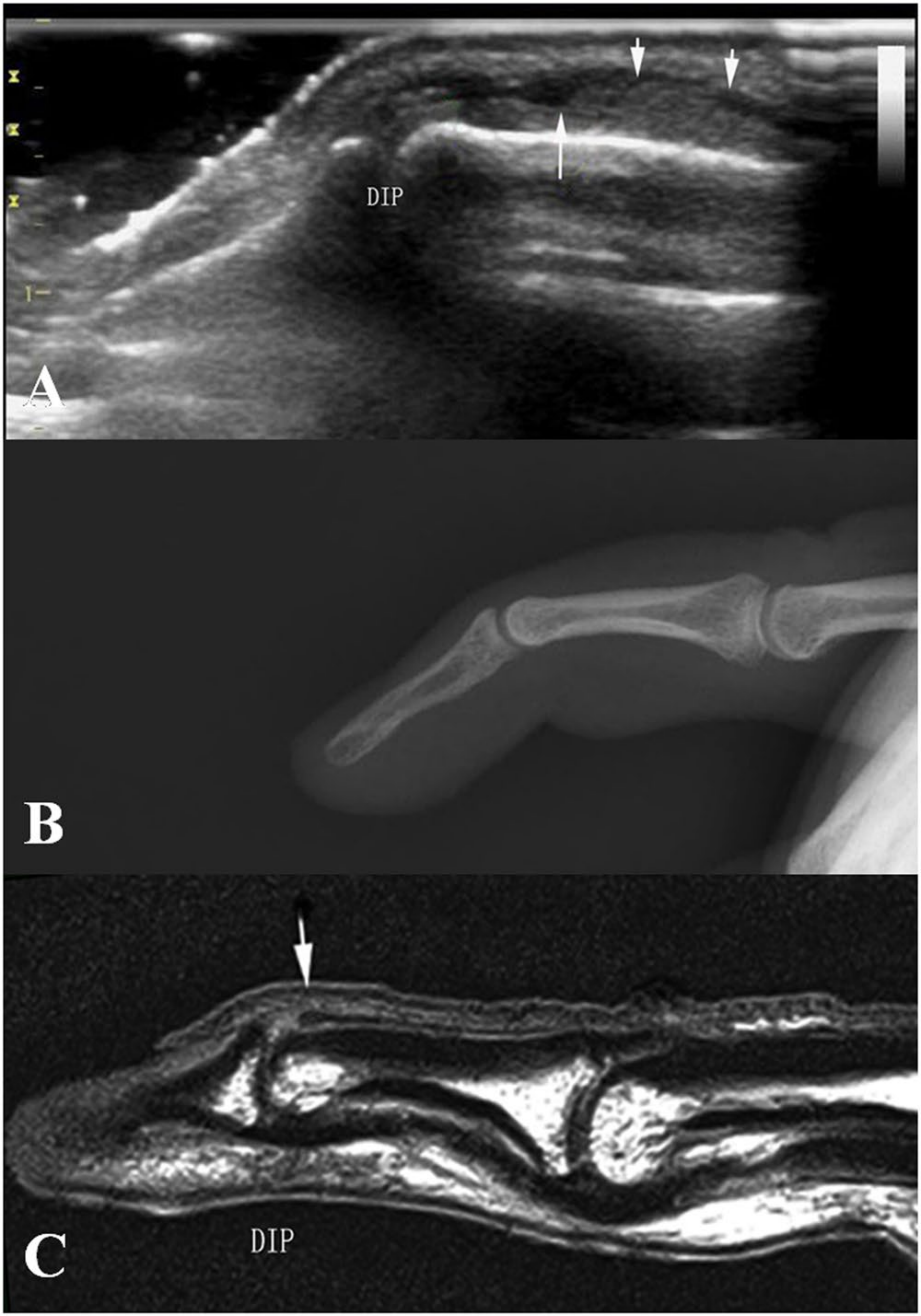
Type B mallet finger injury. High frequency ultrasonography, X-ray and MRI images of type B mallet finger. Figure 3A: The retracted extensor tendon (short arrow), the distal end of extensor tendon (long arrow); Fig. 3B: X-ray image of mallet finger injury. Figure 3C: the retracted extensor tendon (arrow).
